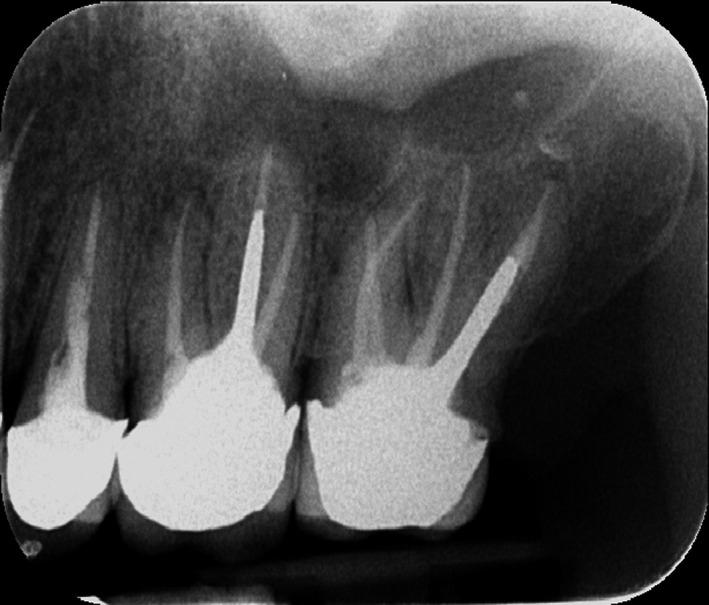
Postoperative Radiograph after Place Cast Post And Coronal Restoration Using Zirconia Crown
